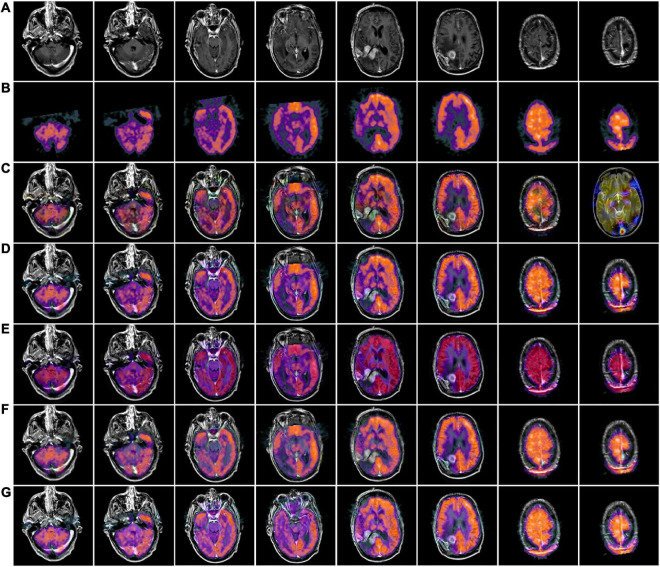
MRI-PET fusion images obtained by five methods in Paras1. (A) Magnetic resonance imaging (MRI) source image; (B) positron emission tomography (PET) source image; (C) nonsubsampled contourlet (NSCT) fusion result; (D) non-subsampled shear wave transform (NSST) fusion result; (E) guided filter ng fusion (GFF) fusion result; (F) Laplacian redecomposition (ReLP) fusion result; (G) fusion result of the proposed method.
